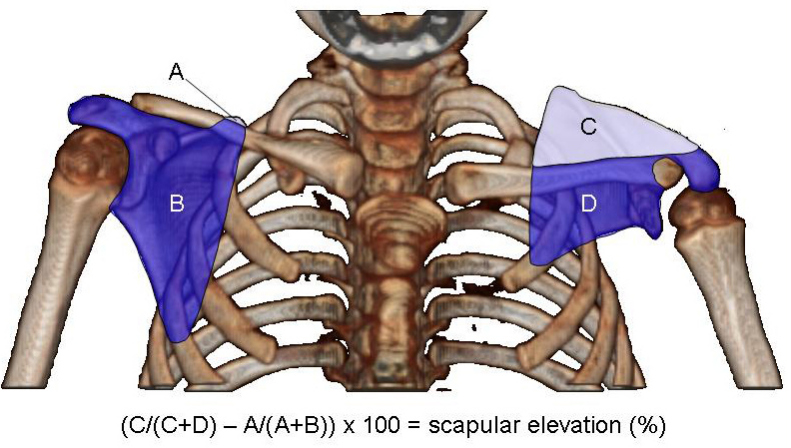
Measuring scapular elevation to quantitate the extent of the SHEAR deformity. A 3D-reconstruction of axial bilateral CT images rotated into the anterior view is used to measure scapular elevation. The area of each portion of both scapulas is measured as indicated (areas A-D). The area above the scapula is divided by the total scapular area and corrected for rotational artifacts by subtraction of the unaffected side from the affected side before multiplying by 100 to obtain percent elevation. Shown here is the CT for patient 1 with 37% scapular elevation.
